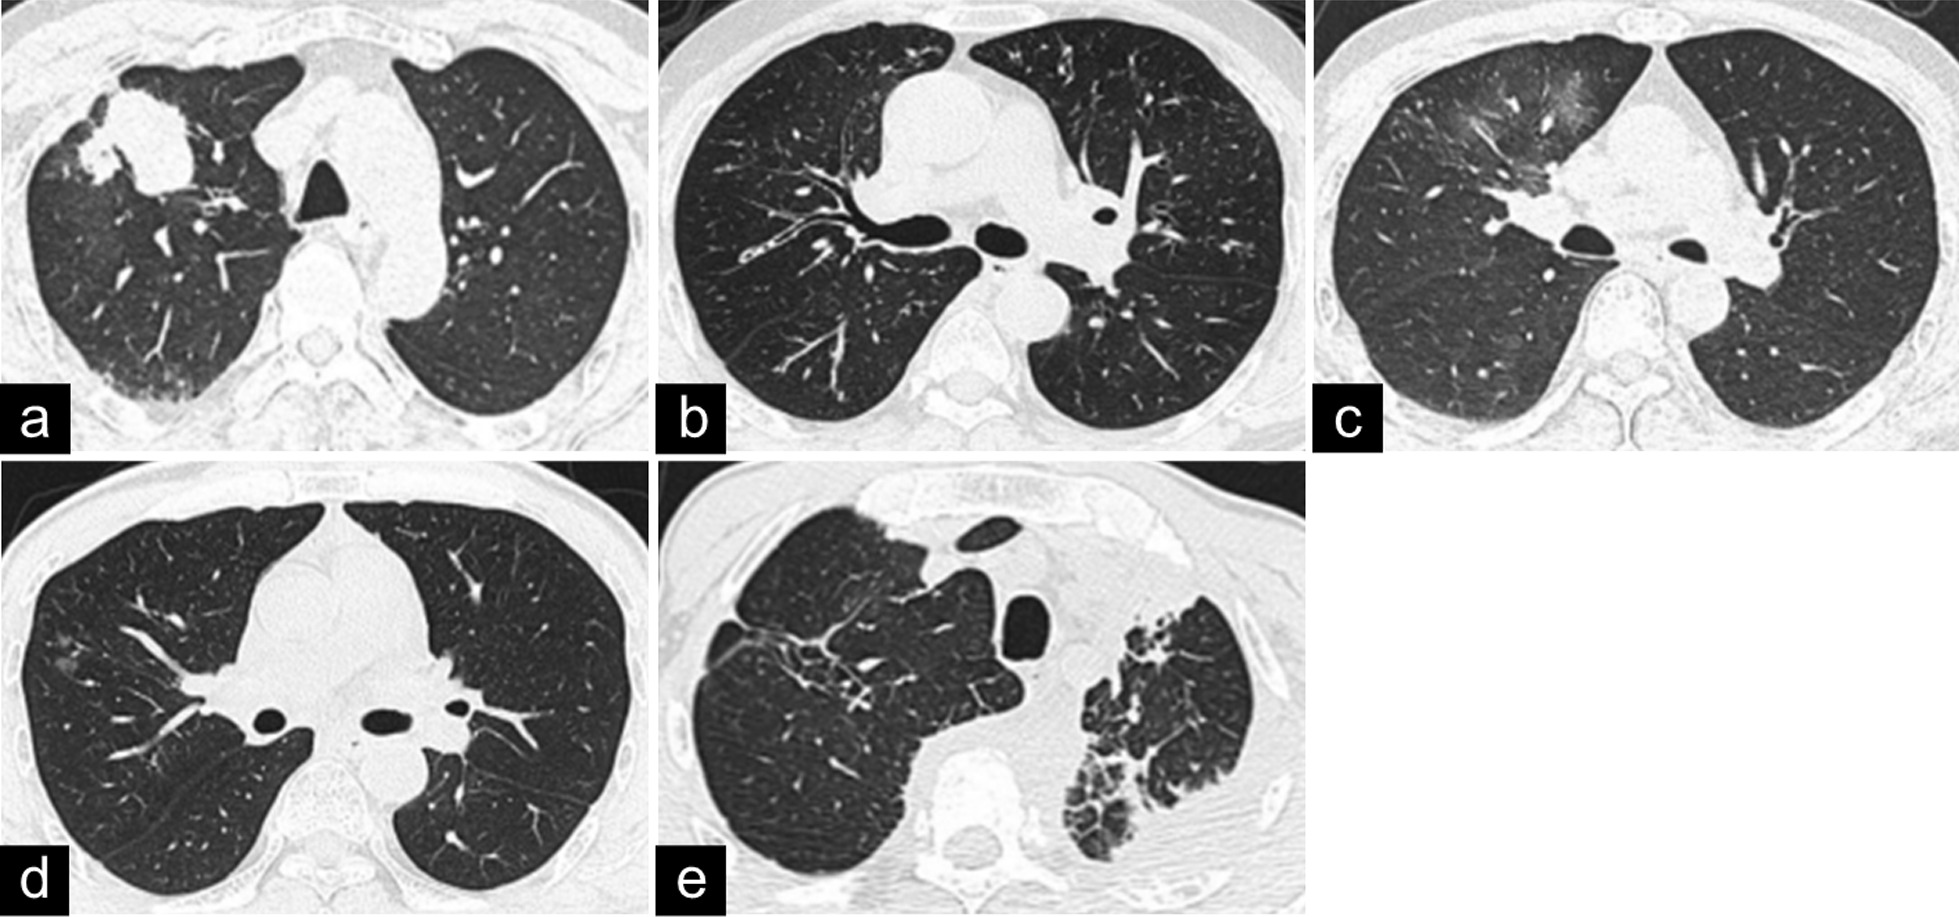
Radiological manifestations of IgG4-related lung disease on chest computed tomography. a A mass with a spiculated margin and pleural indentation can be observed in the right lung. b Thickening of the bronchovascular bundles and bronchiectasis are noted. c Diffuse ground-glass opacities can be observed in the right lung. d Round-shaped ground-glass opacities can be observed in the right lung. e Alveolar consolidation and thickening of the interlobular septa can be observed in the left lung
